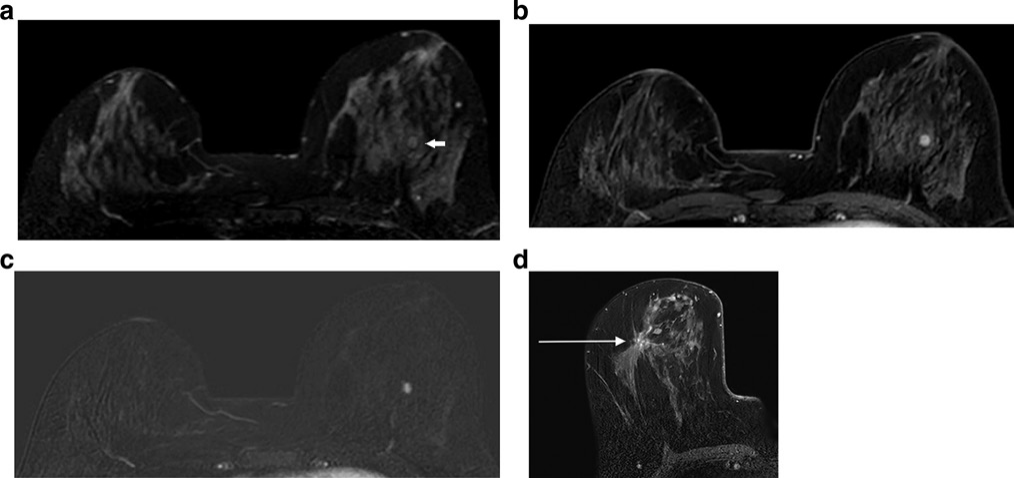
Radial scar. (a), (b) and (c) show a radial scar in the central left breast manifested on breast MRI as a small well-circumscribed enhancing mass (white arrow), that is barely visible on fat suppressed T2W imaging (a). The radial scar is clearly seen on a post contrast, fat suppressed T1W image from the dynamic series (b) and its enhancement is confirmed on the equivalent subtracted slice (c). (d) shows a different case with a typical appearance of a radial scar. The image is a T1W delayed high resolution image, kindly provided by Sarah Vinnicombe, MRCP FRCR, Consultant Radiologist, Cheltenham General Hospital, Gloucestershire Hospitals NHS Foundation Trust, and shows distortion with delayed enhancement within the central right breast (white arrow).
